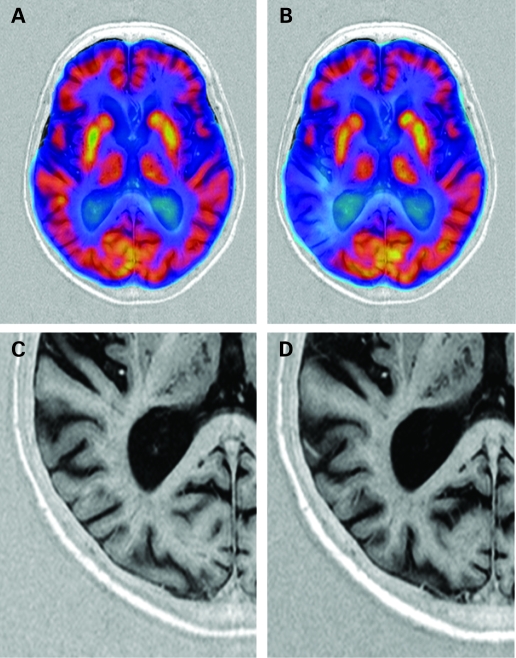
A–D. Baseline (A) and 52-week (B) positron emission tomography (PET) scans registered on MRI in an 85-year-old female study participant who experienced a focal, almost complete loss of glucose utilisation in the right temporoparietal region. Glucose metabolism in other brain regions remained almost unchanged from baseline. The baseline scan demonstrated the typical symmetric temporo-parietal hypometabolism of patients with Alzheimer disease (AD). The coregistered T_1_-weighted MRI scans show little, if any, sulcal enlargement in the area of metabolic loss in this patient between baseline (C) and follow-up (D).
